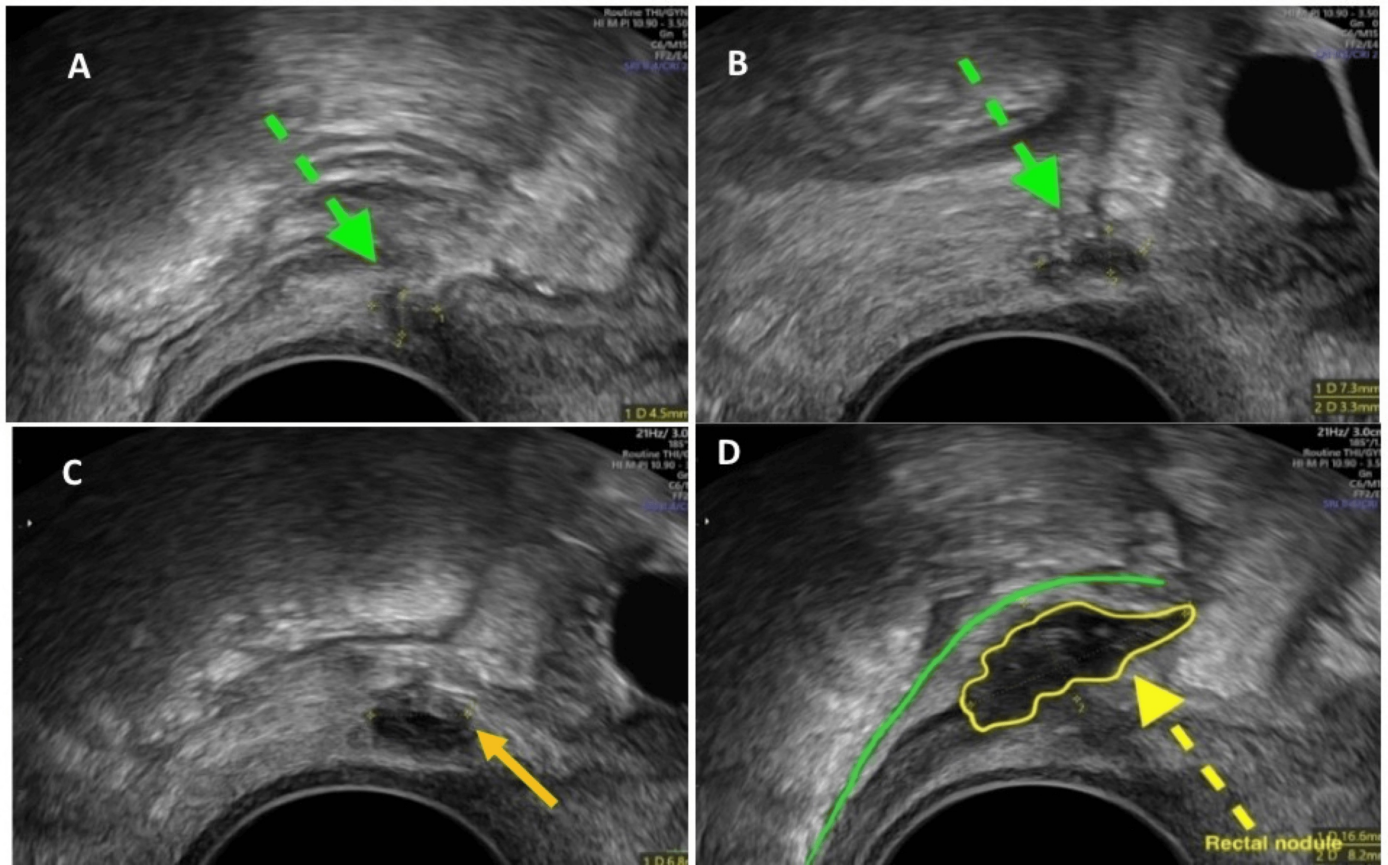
A) TVS ultrasound image showing an endometriotic nodule in the right uterosacral ligament (green dotted arrow). B) TVS ultrasound image showing an endometriotic nodule in the left uterosacral ligament (green dotted arrow). C) Endometriosis nodule in the right parametrium (yellow arrow). D) Endometriosis nodule along the anterior wall of the upper rectum (yellow arrow, green line outlines the mucosa of the rectum). TVS: transvaginal
